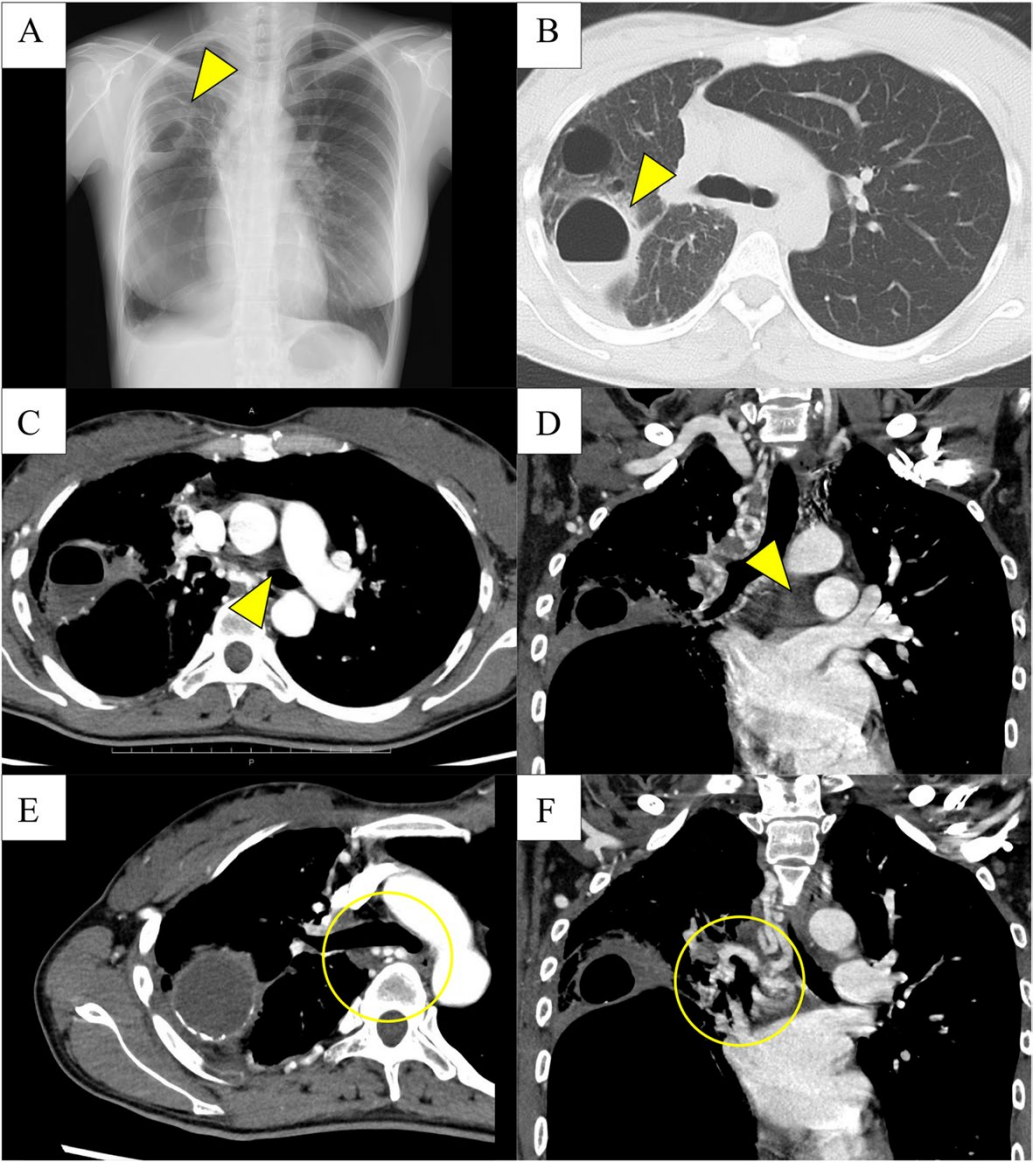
Perioperative imaging. A Chest radiography showing the fluid collection in one of the right lung bulla. B Computed tomography (CT) showing the cavity with the fluid collection and inflammatory changes around the cavity, suggesting an infection. C, D A contrast-enhanced CT scan confirming the absence of the right pulmonary artery. E, F Collateral arteries are shown to have developed from the bronchial arteries to the aortic arch
